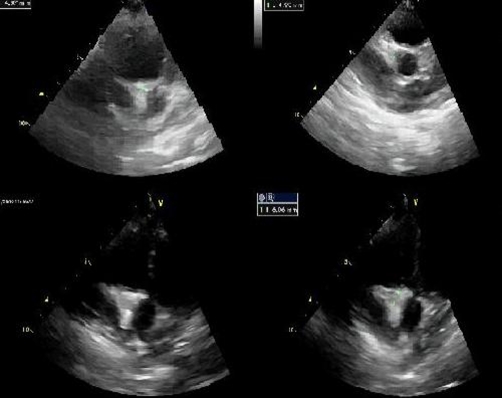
Echocardiographic images showing right coronary artery dilatation.
